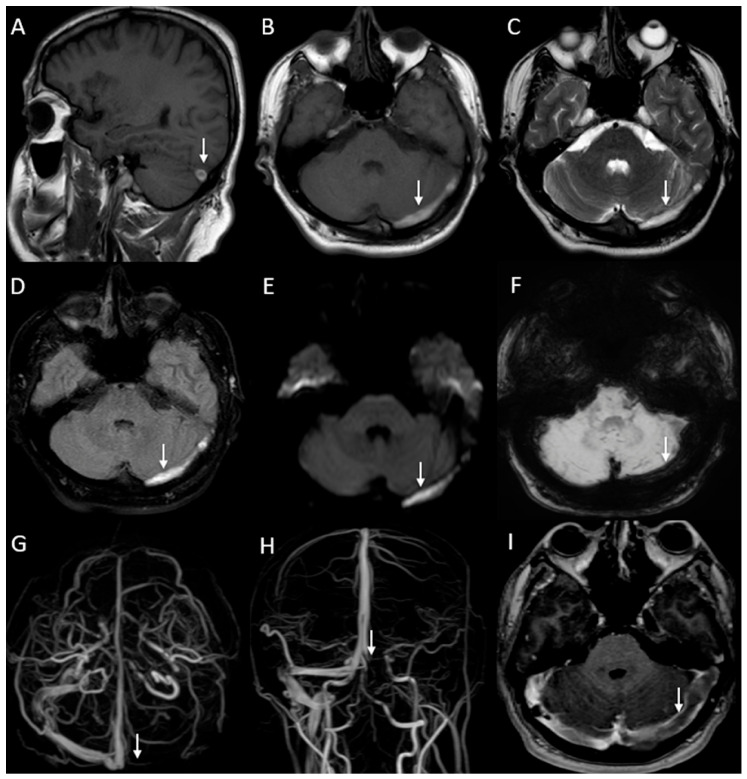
MR imaging signs of dural venous sinus thrombosis in a representative case. (A), Hyperintense signal in the left transverse sinus on sagittal T1WI. (B), Hyperintense signal in the left transverse sinus on axial T1WI. (C), Loss of flow void in the left transverse sinus on axial T2WI. (D), Hyperintense signal in the left transverse sinus on FLAIR. (E), Hyperintense signal in the left transverse sinus on DWI. (F), Blooming artifacts in the left transverse sinus on SWI. (G,H), Filling defect in the left transverse/sigmoid sinus on MIP-CE-MRV. (I), Filling defect in the left transverse/sigmoid sinus on CE-3D T1WI. The thrombosed sinus is indicated by white arrows in all images.
